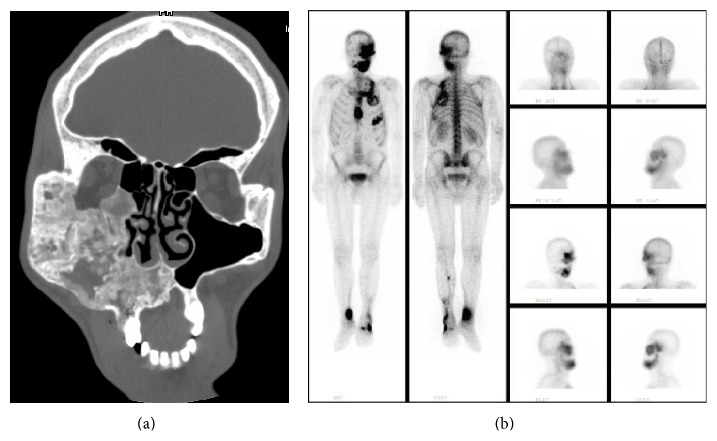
Radiologic and scintigraphic images of patient 5. (a) Cranial CT image. (b) Scintigraphic image.
